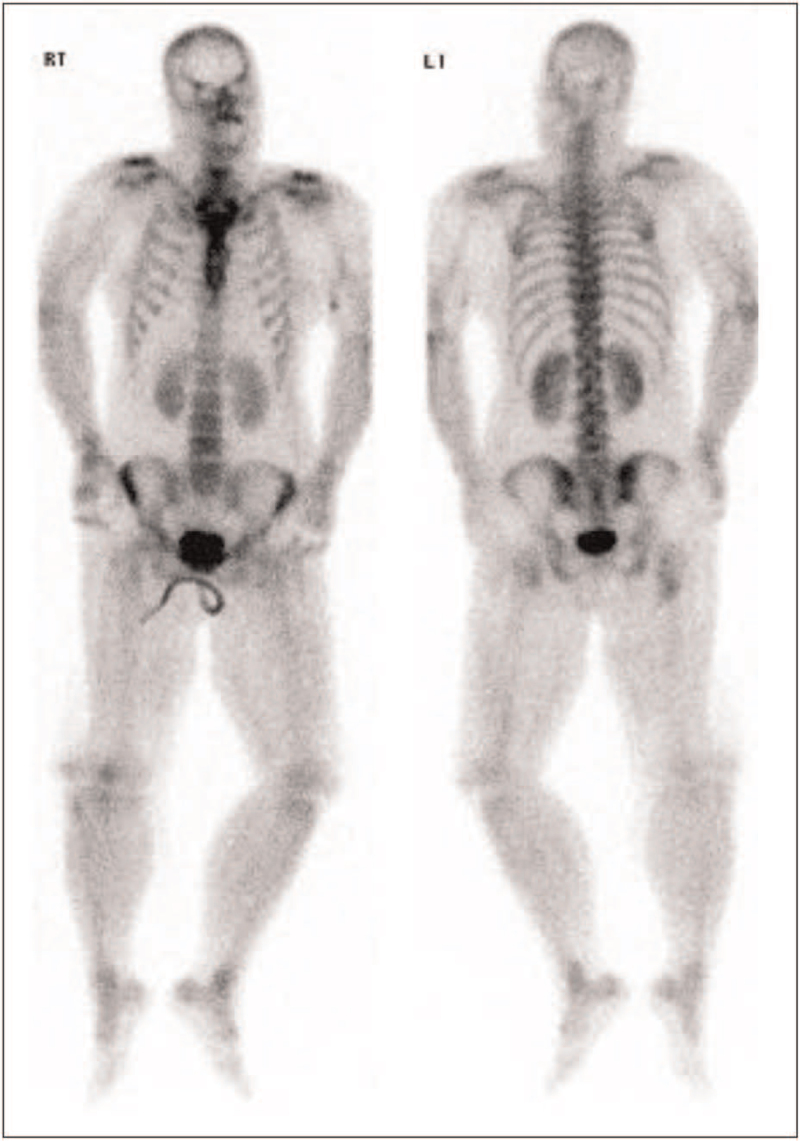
A ^99m^Tc-HDP whole-body bone scintigraphy on the 5th hospital day showed diffusely increased soft tissue uptake of radiotracer in the both upper and lower extremities.
